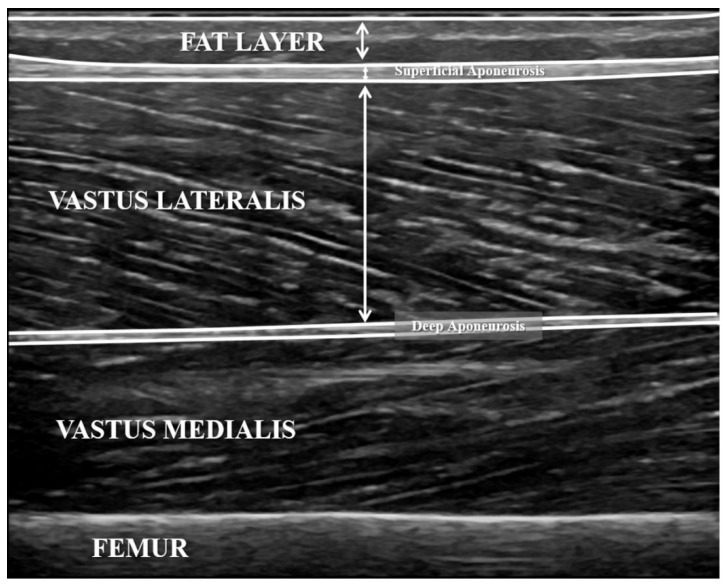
B-mode ultrasound exam of vastus lateralis. Vastus lateralis thickness was calculated as the distance in mm between the deep edge of the superficial aponeurosis and the superficial edge of the deep aponeurosis; adipose tissue thickness was calculated as the distance in mm between the skin and the superficial edge of the superficial aponeurosis; superficial connective tissue thickness was calculated as the distance in mm between the deep edge and the superficial edge of the superficial aponeurosis.
